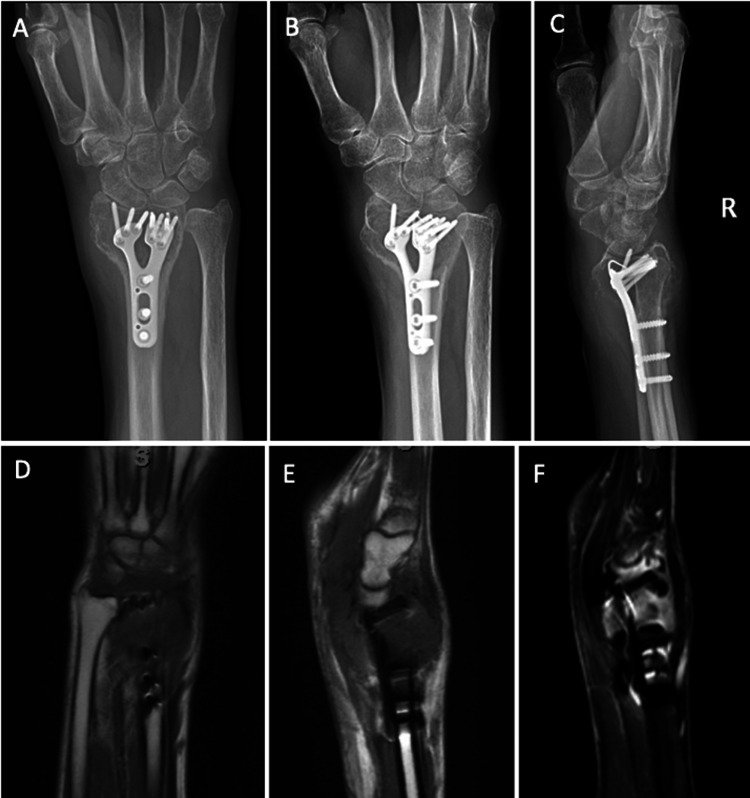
Follow-up X-rays and MRI A-C) Five-month postoperative anteroposterior, oblique, and lateral X-rays views of the right wrist: enlarged metaphyseal lucency with a dorsal cortical appearing breach, intact hardware, and continued loss of radial height. D-F) T1 coronal, T1 sagittal, and FS T2 sagittal MRI sequences showing enlarged, dorsal metaphyseal lesion T1 hypointense and T2 hyperintense classic for giant cell.
